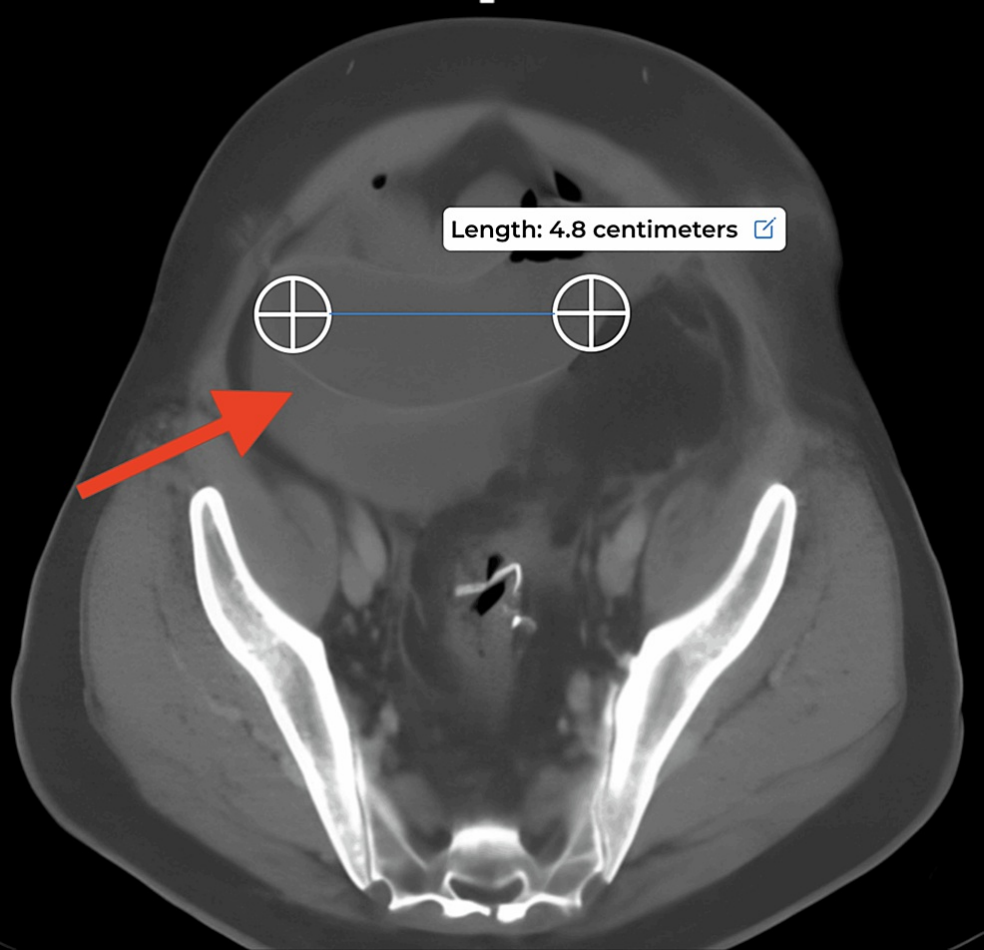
CT Abdomen and Pelvis Multiple dilated loops of the small bowel, measuring up to 4.8 cm, with an apparent transition point in the mid-abdomen.
